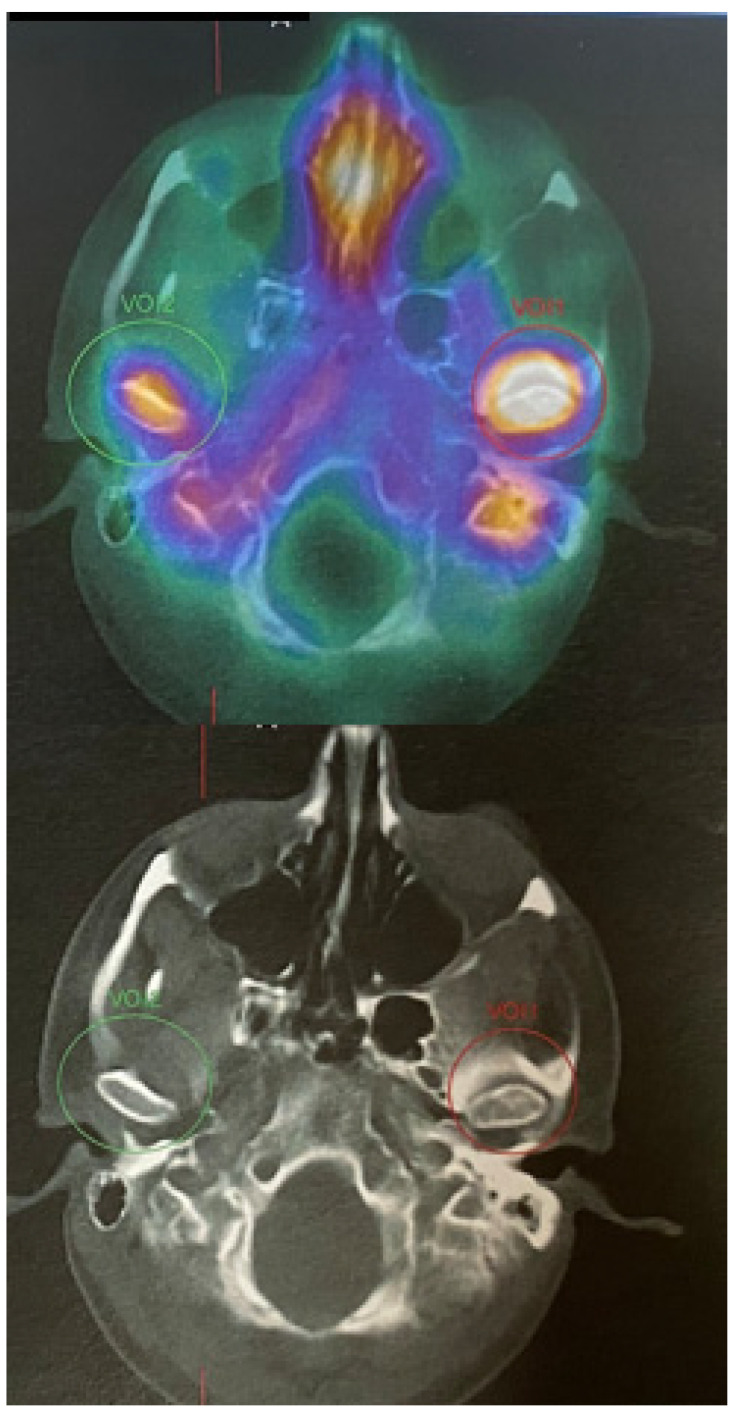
SPECT-CT of the facial skeleton revealed an atypical, extensive, and progressive accumulation of growth in the left mandibular condyle head. The MDP Tc99m (Technetium 99m methylene diphosphonate) is quite important because any accumulation within the affected condyle might either mean inflammation, bone growth, abnormal bone growth, bone tumor, joint overload, some atypical tumors/bone metaplasia, or other findings when the craniofacial skeleton is evaluated. Some authors suggest that the differences in radio uptake of more than 10–15% might suggest growing condylar hyperplasia; however, a full clinical, radiological and SPECT comparison should be drawn at least two times in 4–6 months’ time to compare their features. SPECT-CT alone is not enough to confirm any UCH pathology; therefore, a detailed CT/CBCT/LDCT evaluation and clinical patient examination can greatly influence the future final identification of the disease. Many authors emphasize that SPECT is a very important diagnostic tool and should be always performed in cases of any skeletal asymmetries, especially mandibular asymmetry [4,5]. Abbreviations: UCH—unilateral condylar hyperplasia; CT/LDCT—computed tomography/low-dose computed tomography; green circle–negative growth; red circle–proggresive bone growth in SPECT.
